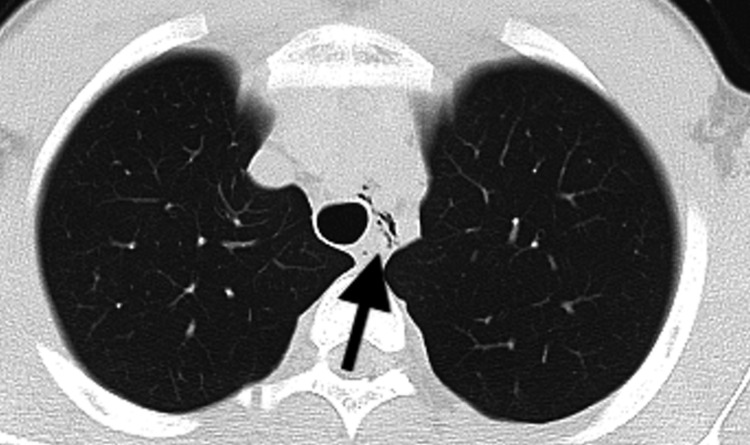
Chest CT images showing pneumomediastinum surrounding the left main bronchus (third episode) CT: computed tomography
